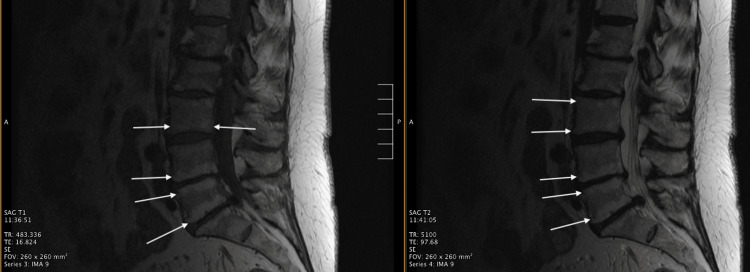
MRI sagittal T1 (left) and T2 (right)-weighted images demonstrating (white arrows) a mix of both Type 1 and Type 2 Modic endplate changes on the L2, L3, L4, L5, and S1 vertebral bodies. Note that we only performed the procedure at L3, L4, and L5.
